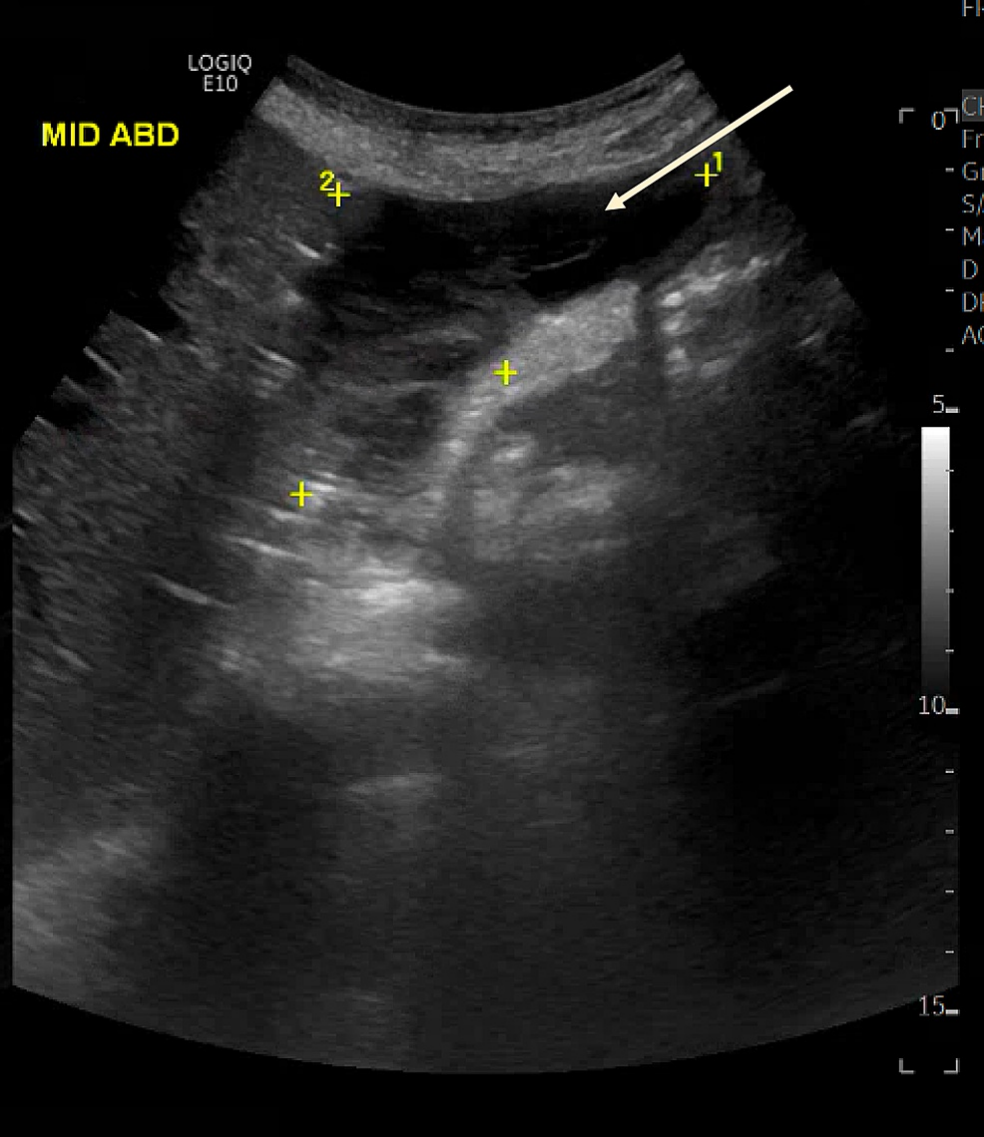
Ultrasound showing peri-hepatic fluid collection
